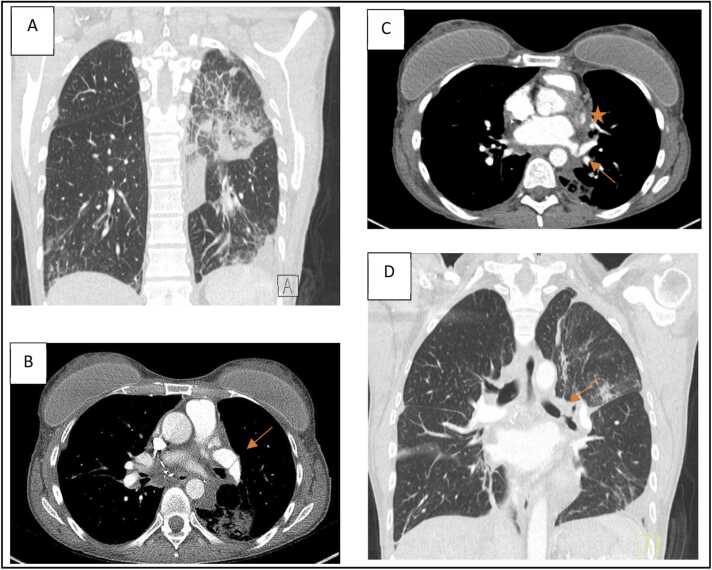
Chest computed tomography scan 20 days postoperatively. A: Infarction of left upper lobe; B: Kinking of the left main pulmonary artery (arrow); C: Occlusion of the left superior pulmonary vein (star) and kinking of the left inferior pulmonary vein (arrow); D: Stenosis at the left bronchial anastomosis (arrow).
